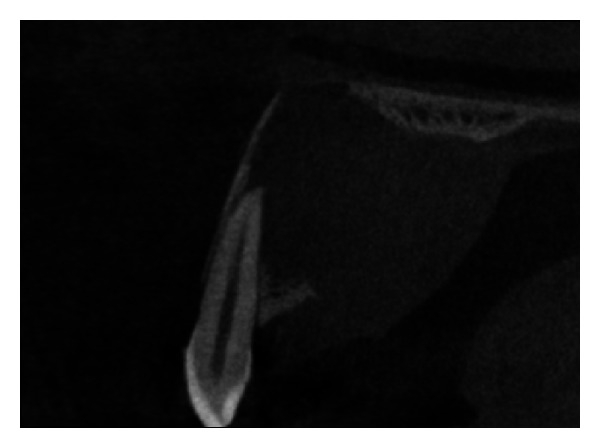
Pre-operative CBCT image in sagittal plane.
